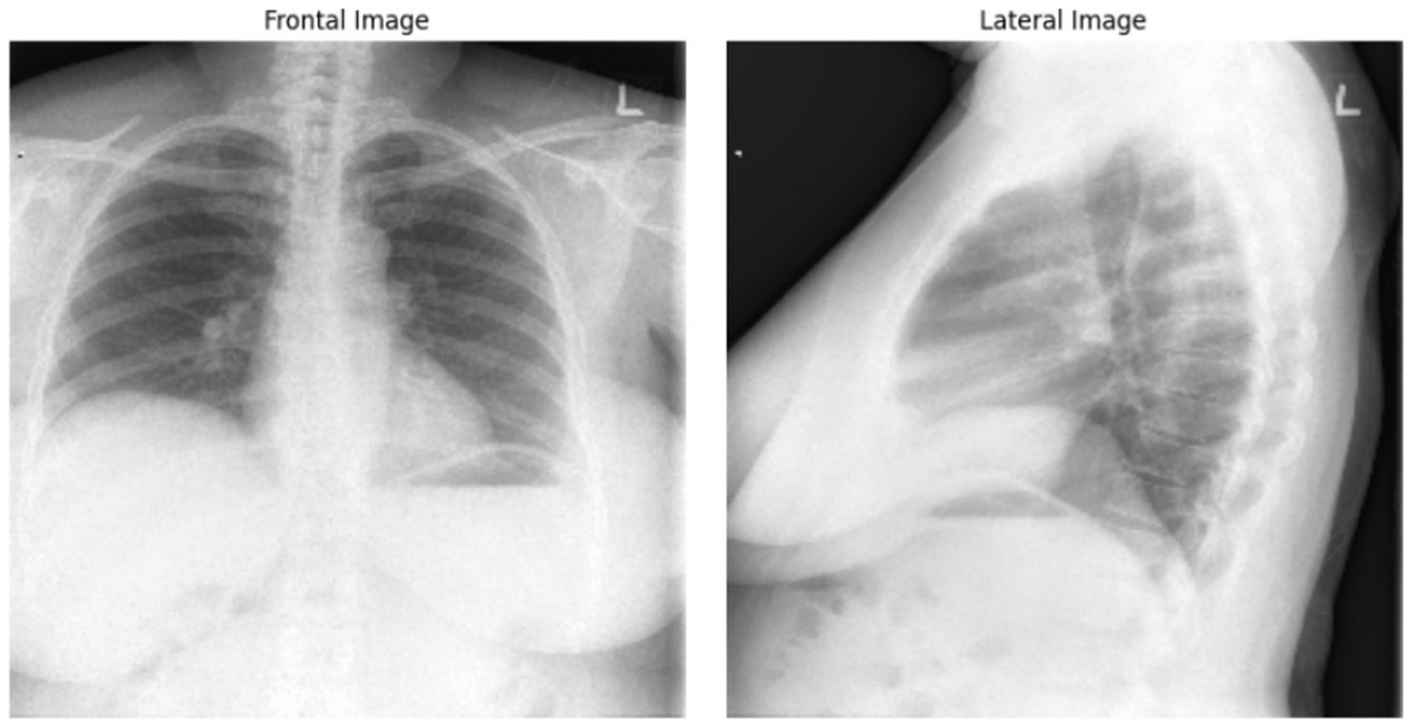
Frontal and lateral view of Chest X-ray images. The example from “Chest X-rays” dataset of Indiana University.
